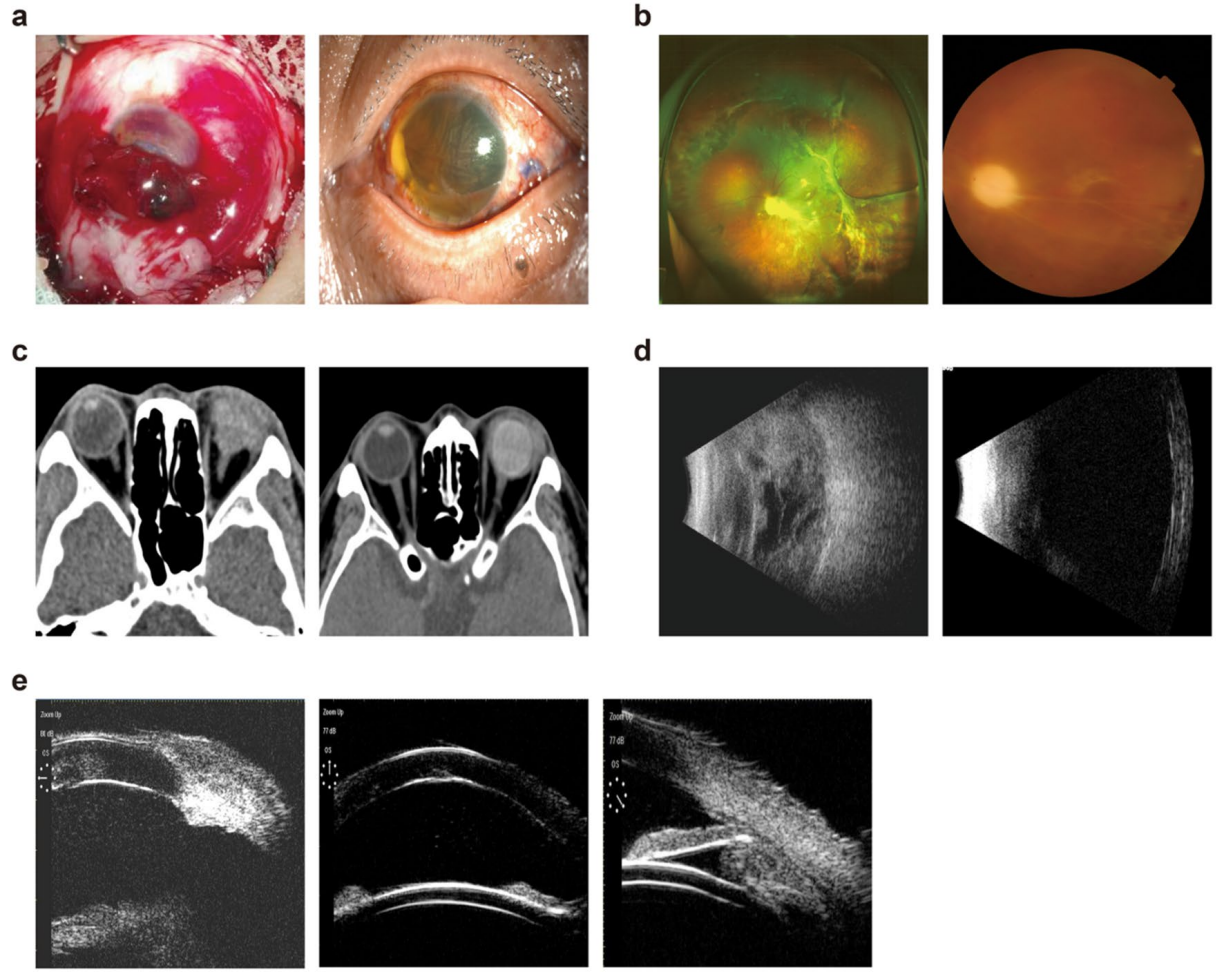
Images of before/after the FCVB implantation. (a) Conditions of a cornea with inferior limbal rupture before/after the operation (b) Fundus photography after FCVB implantation (c) Orbital CT images before and after FCVB implantation (d) B-scan images before and after FCVB implantation (e) UBM images before and after FCVB implantation
